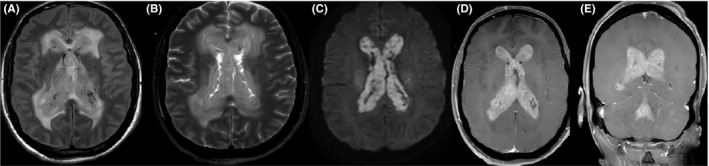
Intraventricular melanoma metastasis. A and B, axial FLAIR and T2 sequences demonstrate mild dilation of the ventricular system with near complete obliteration of the lateral ventricles by a heterogeneous and mainly hyperintense soft tissue mass. In addition, there is significant periventricular FLAIR/T2 signal hyperintensity consistent with subependymal interstitial edema. C, Axial diffusion‐weighted imaging (DWI) demonstrates a soft tissue mass within the ventricular system, which shows marked diffusion restriction. (D) Axial and (E) coronal T1 postcontrast sequences show near‐complete obliteration of the ventricular system with a hyperenhancing soft tissue mass that is compatible with extensive intraventricular metastatic melanoma
